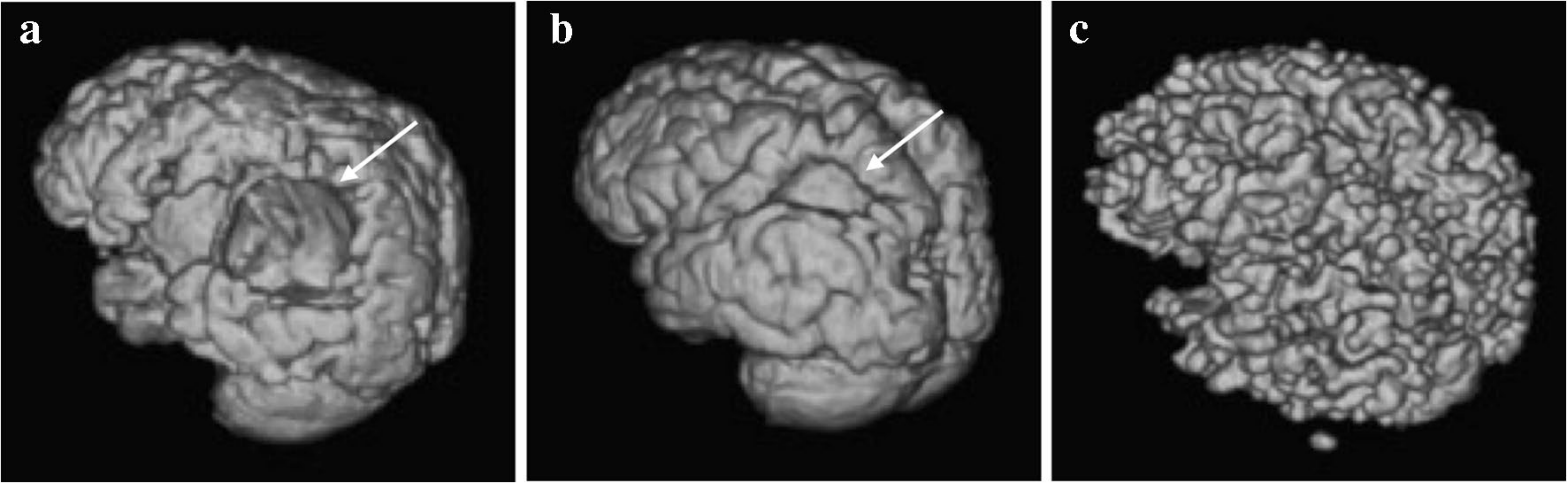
Standard tessellation language surface model examples depicting unsuccessful segmentation of an MRI of the brain in a 5-year-and-2-month-old boy with default settings for (a) Computational Anatomy Toolbox version 12, (b) Statistical Parametric Mapping version 12, and (c) MRTool segmentations. a Shows a large open hole in the volume (*arrow*). b Compared to (a), this image shows a  smaller hole in the volume (*arrow*). c There is diffuse exclusion of outer gray matter (incorrect segmentation of the gray matter) in the entire volume, as demonstrated by the extreme irregular sulcal pattern when compared to the models in (a) and (b)
